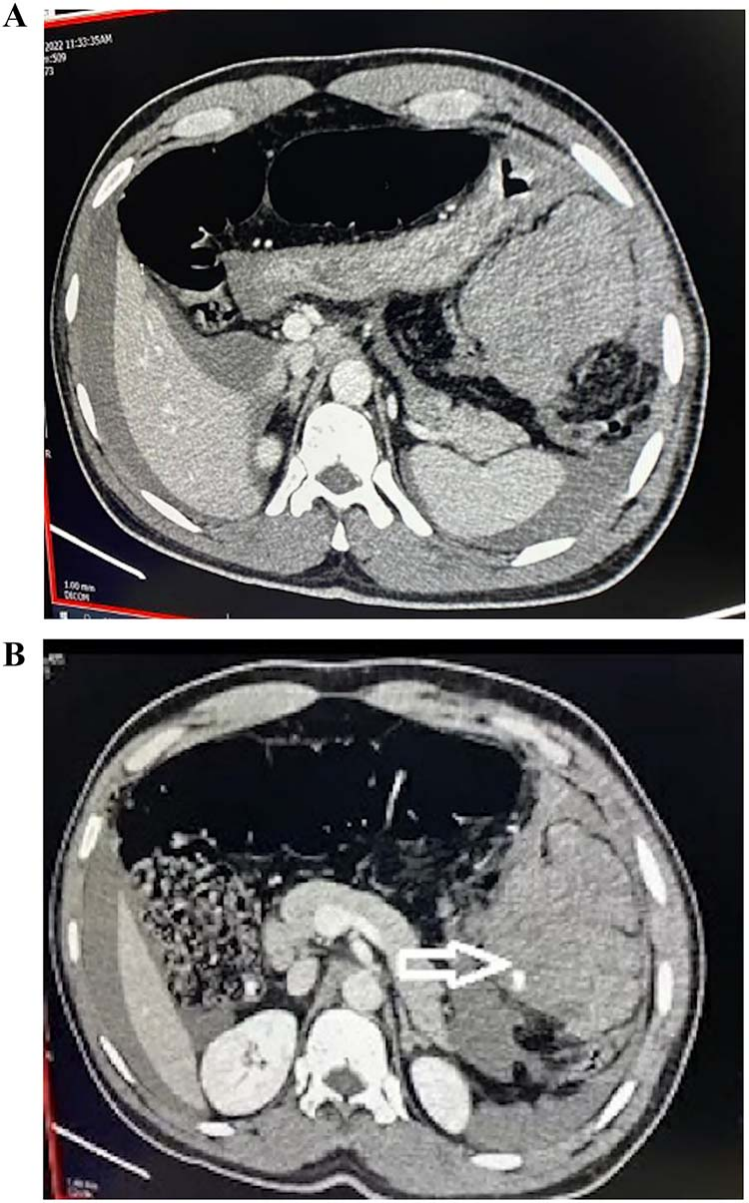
(A, B) CT abdomen and pelvis with IV contrast demonstrating hyperdense area of blood density suggestive of hematoma with eccentric focal intrinsic extravasation of contrast at left hypochondrium.
